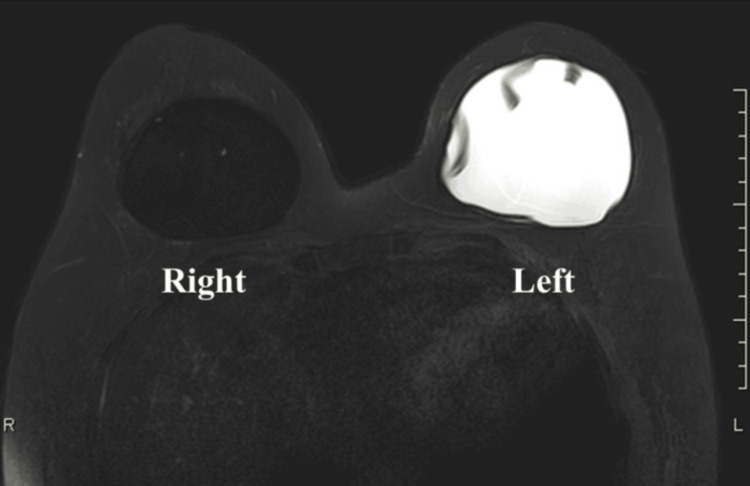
T2-weighted MRI of the breasts The left silicone implant shows irregular margins and internal linear high-signal structures on T2-weighted imaging, suggestive of intracapsular rupture. The right breast appears normal.
